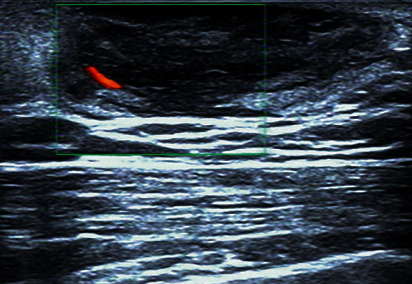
The typical ultrasound image of dermatofibrosarcoma protuberans of the breast. Note: ultrasound shows a well-defined hypoechoic mass in the subcutaneous tissue with a slightly hyperechoic surrounding area. Increased internal vascularity of the lesion is demonstrated by color Doppler scanning.
